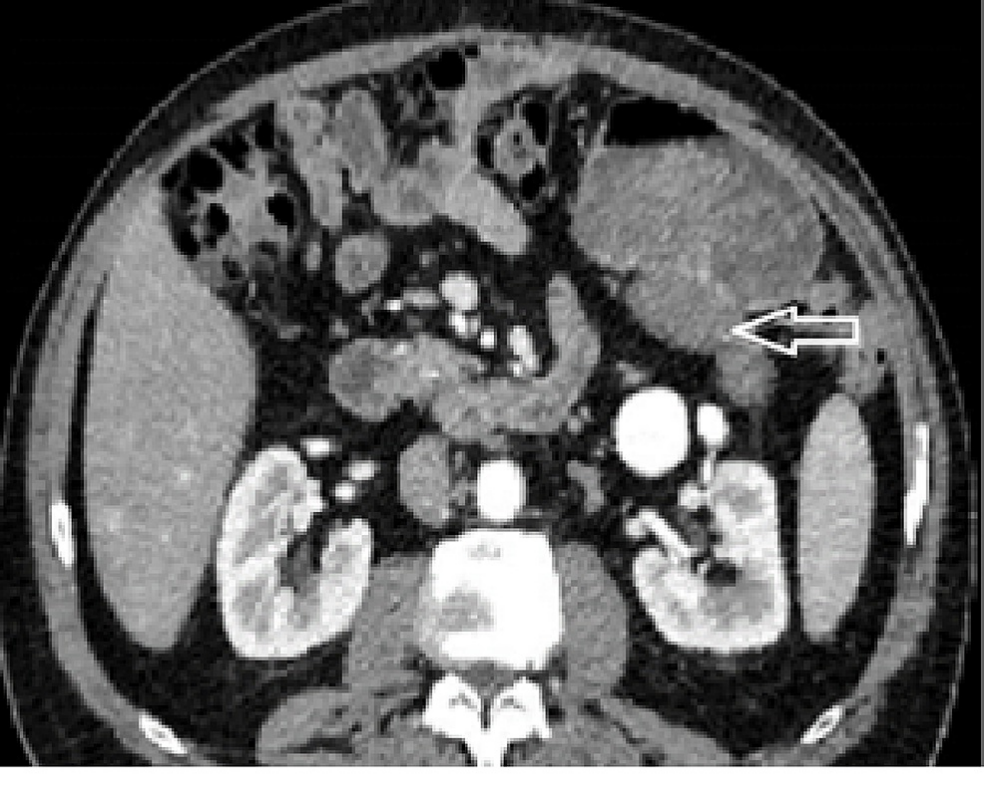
Case 2. Contrast-enhanced abdominal CT scan The white arrow points to a homogenous mass on the posterior wall of the stomach.
